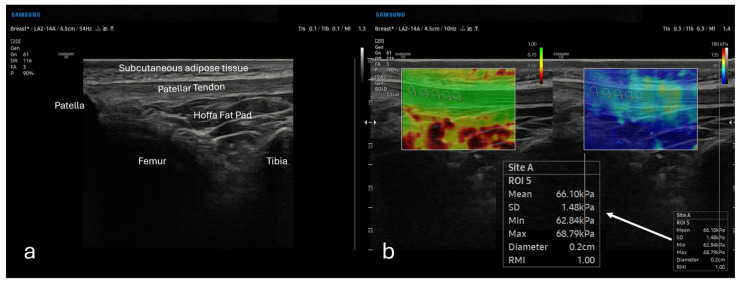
Ultrasound and shear-wave elastography assessment of the patellar tendon. (a) Longitudinal grayscale ultrasonographic image obtained at the anterior knee, demonstrating the patellar tendon and adjacent anatomical landmarks, including the patella, subcutaneous adipose tissue, Hoffa fat pad, femur, and tibia. (b) Shear-wave elastography (SWE) image of the patellar tendon with the elastography map overlaid on the B-mode image. Five 0.2 cm circular regions of interest (ROIs) placed along the tendon are shown for quantitative stiffness measurement (kPa). The arrow indicates the magnified quantitative SWE output (example ROI measurement).
